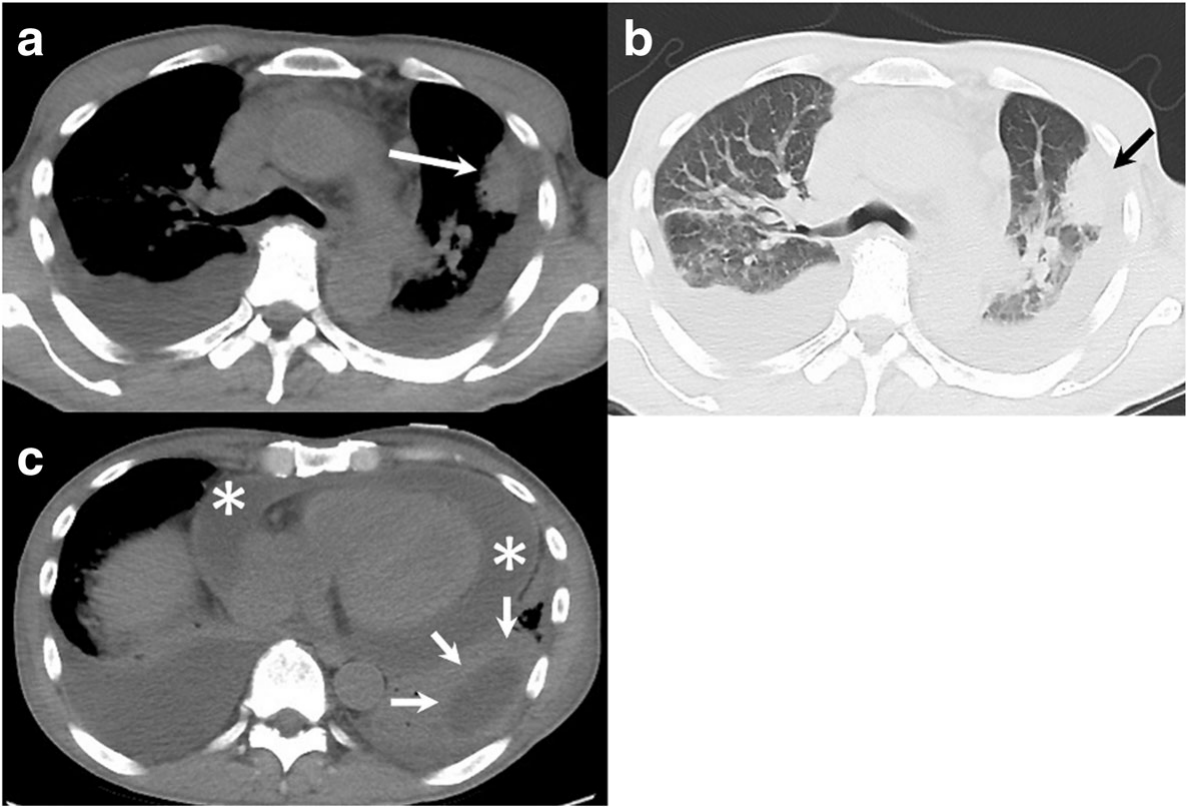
The chest CT scan showed a wedge-shaped and pleural-based mass in the left upper lobe (white arrow on (a), and black arrow on (b)), thick-walled cavitary lesion containing water density in the left lower lobe (white arrows on (c)), bilateral pleural effusions and pericardial effusion (* on (c))
